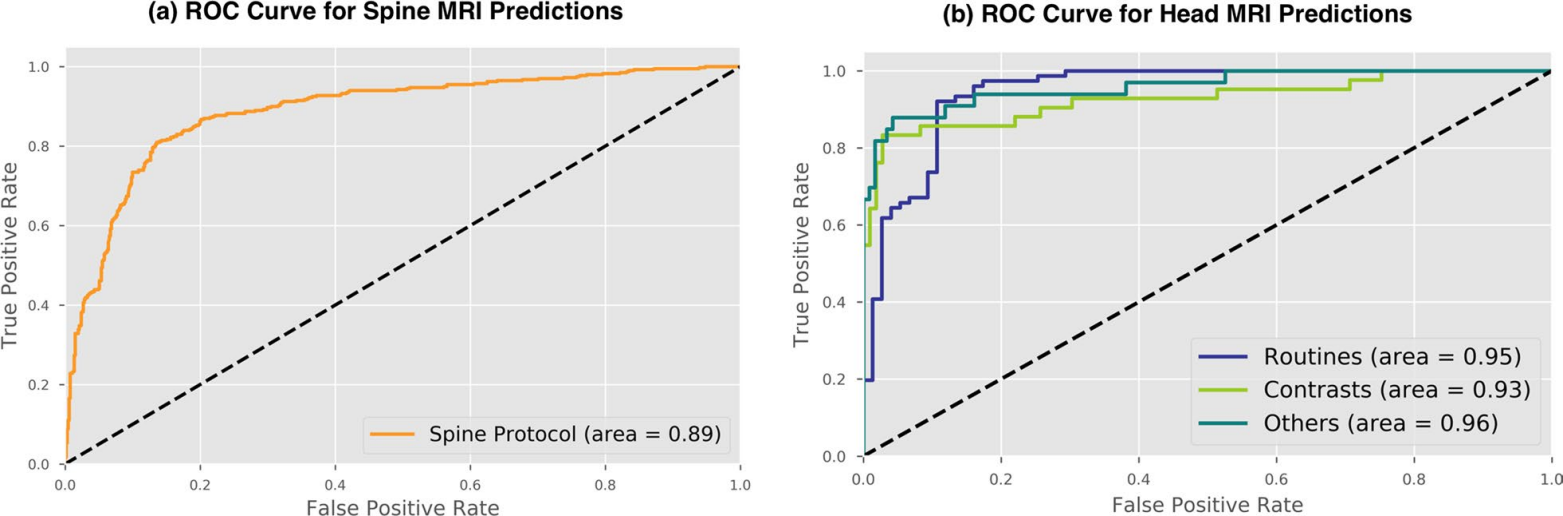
Receiver operating characteristic curves. Receiver Operating Characteristic (ROC) curves for spine and head MRI predictions. a Spine protocol achieved a ROC area under the curve (ROC-AUC) of 0.8873 for differentiating between contrast and non-contrast spine MRI. b Head MRI ROC-AUC was calculated as one vs. other categories for brain MRI routine without contrast (Routines), brain MRI with contrast (Contrasts), and Others (epilepsy, IAC, face, MRA, orbits, sella, and TMJ) and achieved a performance of 0.9463, 0.9284, and 0.9569, respectively
